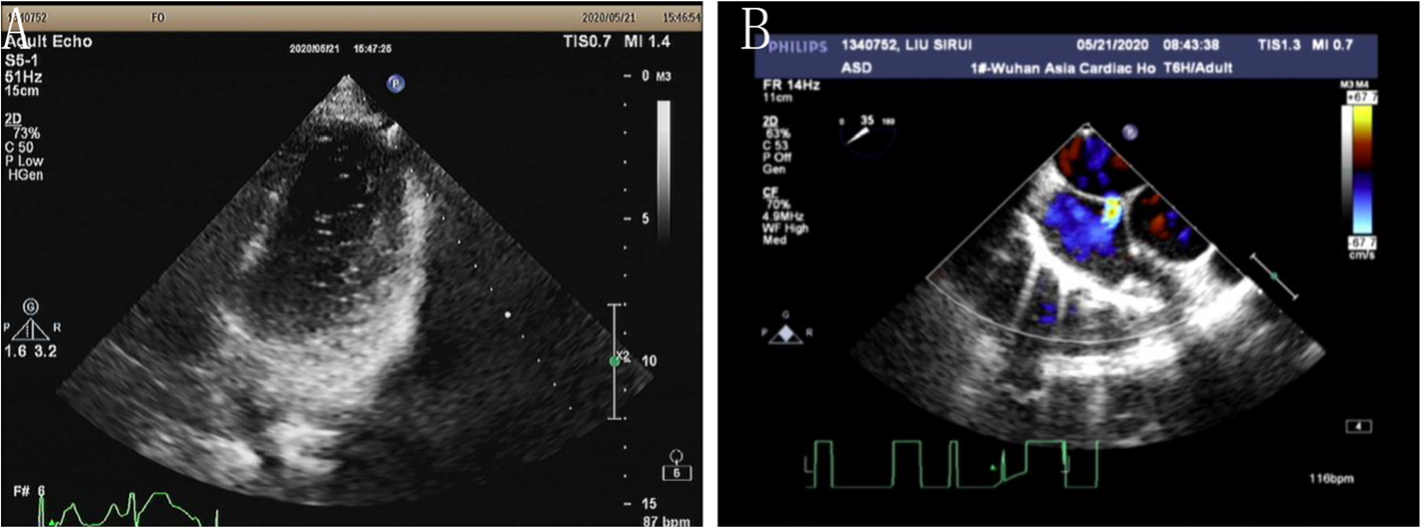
Results for echocardiography of the right heart and TEE before transcatheter closure. a. Echocardiography of the right heart showed a large number of right-to-left shunts at the atrial level. b. TEE showed that echo interruption was seen in the atrial septum, and a left-to-right shunt signal was seen at the atrial level
